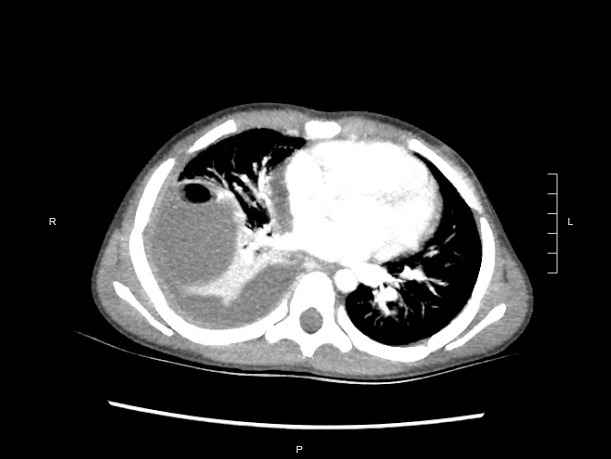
Chest CT at time of the patient’s second presentation at the hospital, demonstrating a right cavitary lung lesion with air-fluid level.
